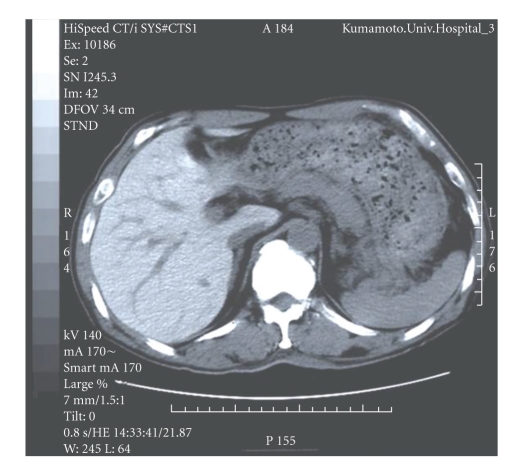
Abdominal computed tomographic scanning through liver and spleen. Note the marked increase in liver density (113 Hounsfield units) compared to the spleen.
